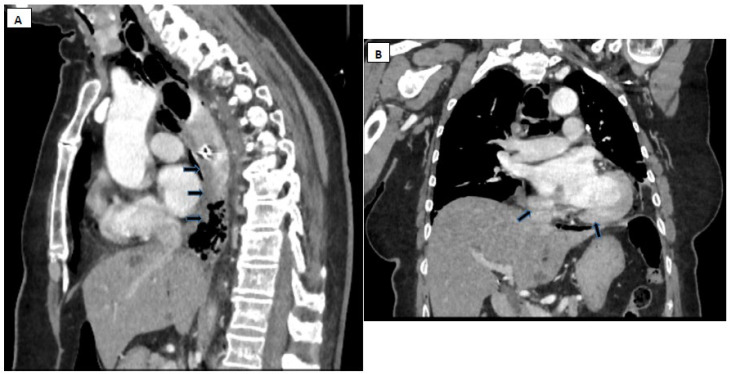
Thoraco-abdominal CT scan with contrast agent, sagittal (A) and frontal (B) sections. Images of significant mediastinal, supradiaphragmatic fluid collection (arrows) through an esophageal tear in the middle and lower thirds.
